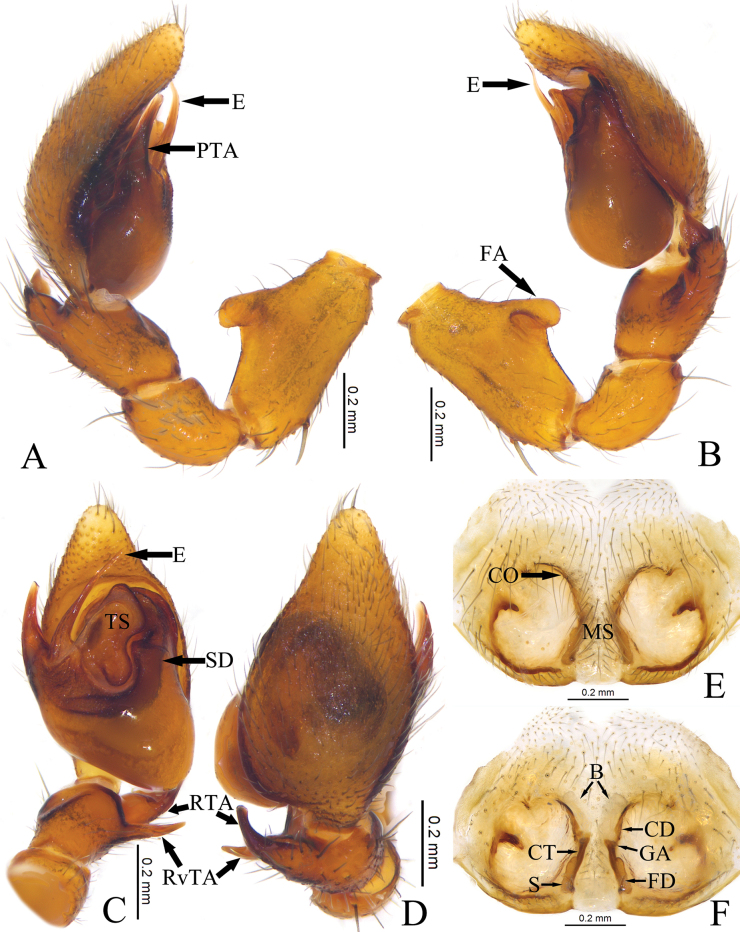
Copulatory organs of *Mutatustianyan* sp. nov. A male left palp, prolateral view B same, retrolateral view C same, ventral view D same, dorsal view E epigyne, ventral view F same, dorsal view. Abbreviations: E—embolus; FA—femoral apophysis; PTA—prolateral tegular apophysis; RTA—retrolateral tibial apophysis; RvTA—retroventral tibial apophysis; SD—sperm duct; TS—tegular sheet; B—bursa; CD—copulatory duct; CO—copulatory opening; CT—connecting tube; FD—fertilization duct; GA—glandular appendage; MS—median septum; S—spermathecae.
